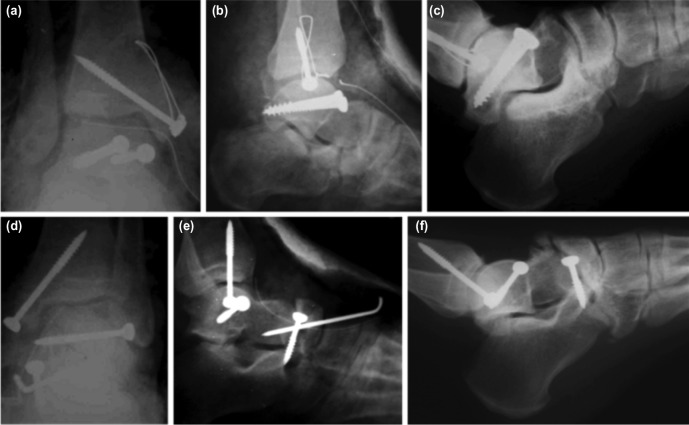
Post-operative radio-imaging of both feet (a) anterior-posterior and (b) lateral radiographs of right ankle showing anatomically reduced right talar fracture with screw fixation. The medial malleolus osteotomy was fixed with one screw and figure-eight wire looping (c) lateral radiographs of the same foot at 36 months follow-up. (d) anterior-posterior. (e) lateral radiographs of the left foot showing anatomic reduction and screw fixation of the left talus posteromedial process fracture, screw fixation of left navicular bone, and Kirschner wire fixation of left talo-navicular joint. (f) lateral radiographic of the same foot at 36 months follow-up.
